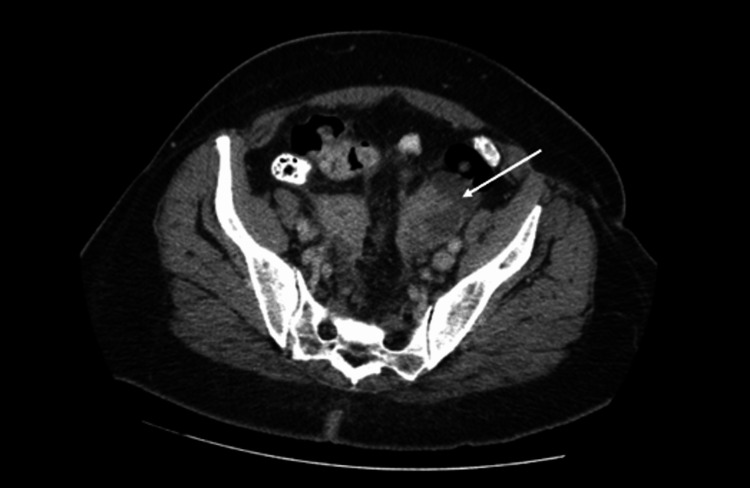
CT scan shows the stricture of the sigmoid colon by a soft tissue mass (transverse).
